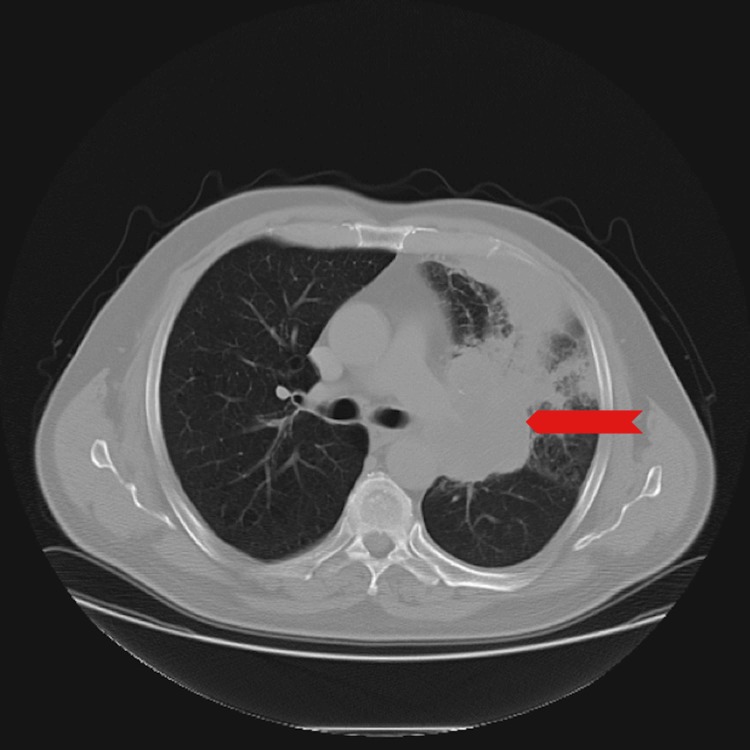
A CT chest showing left hilar mass.
